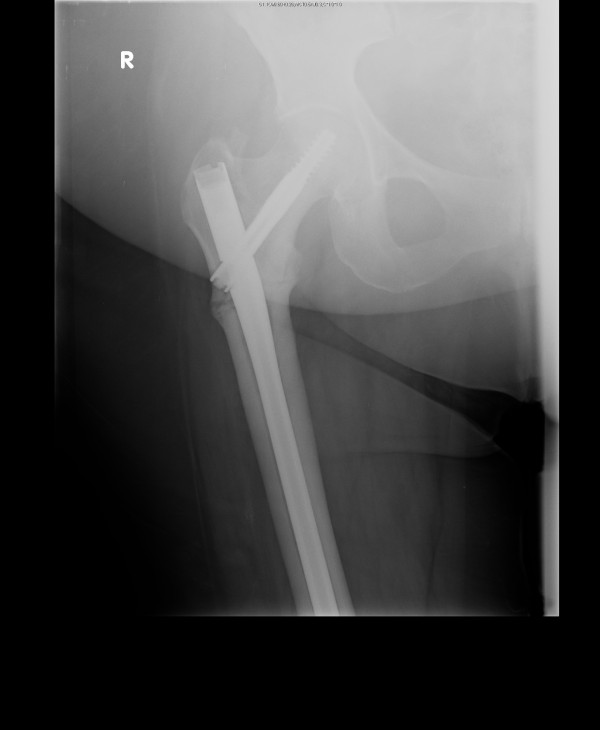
X-ray of the right hip 12 months following Gamma nail insertion with delayed bone healing process.
